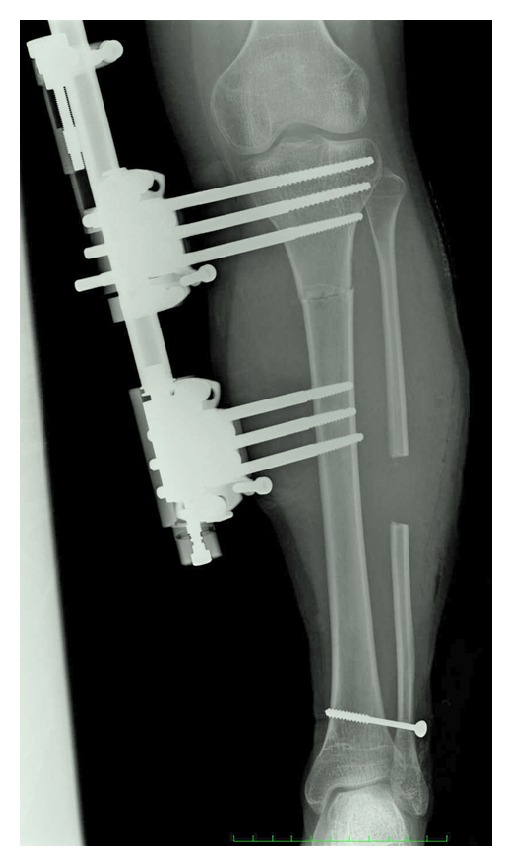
Radiograph of the left tibia after lengthening operation.
